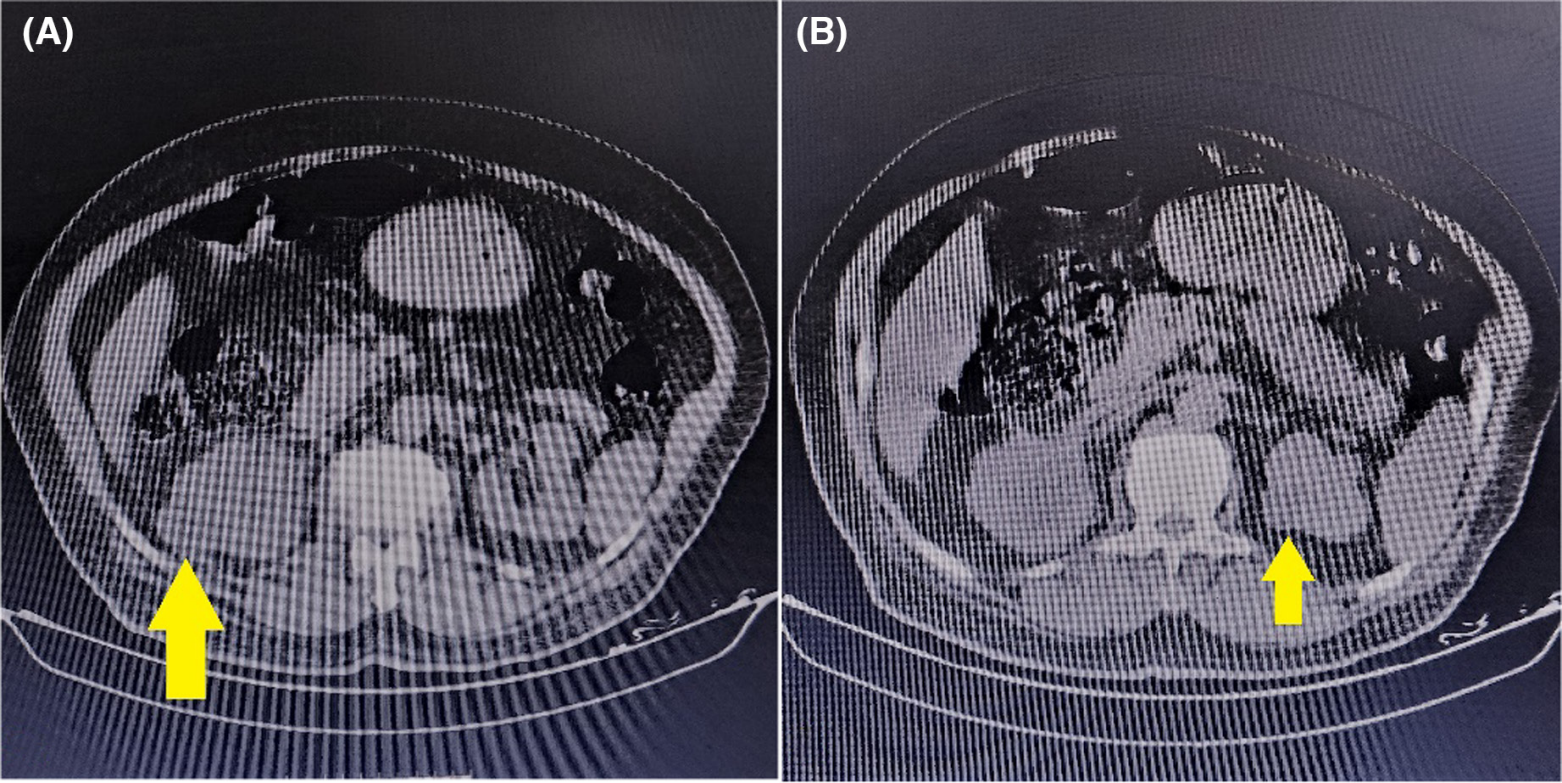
Abdominal CT shows two simple cysts (yellow arrow) in both kidneys and the fungal masses were resolved after a year.
